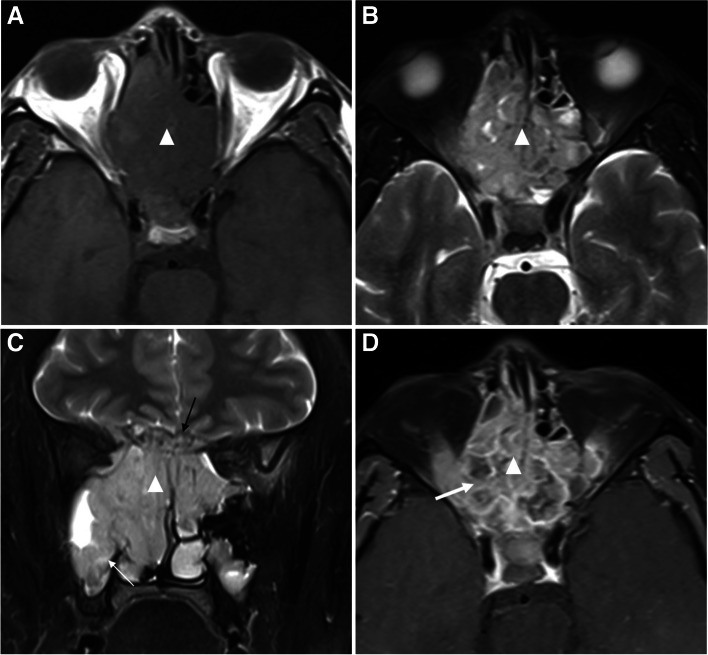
A 36-year-old male patient with ERMS. a T1-weighted, b, c T2-weighted, and d T1-weighted fat-suppressed postcontrast images show an irregular soft tissue mass (triangle) in both ethmoid sinuses. The size of the mass is 5.1 × 5.1 × 5.4 cm^3^ and the margin is ill-defined. Mucosal invasion of maxillary sinus (thin white arrow) and skull involvement (thin black arrow) can be seen. After contrast enhancement, the mass is enhanced moderately and heterogeneously, and botryoid enhancement with multiple small rings (thick arrow) can be seen
